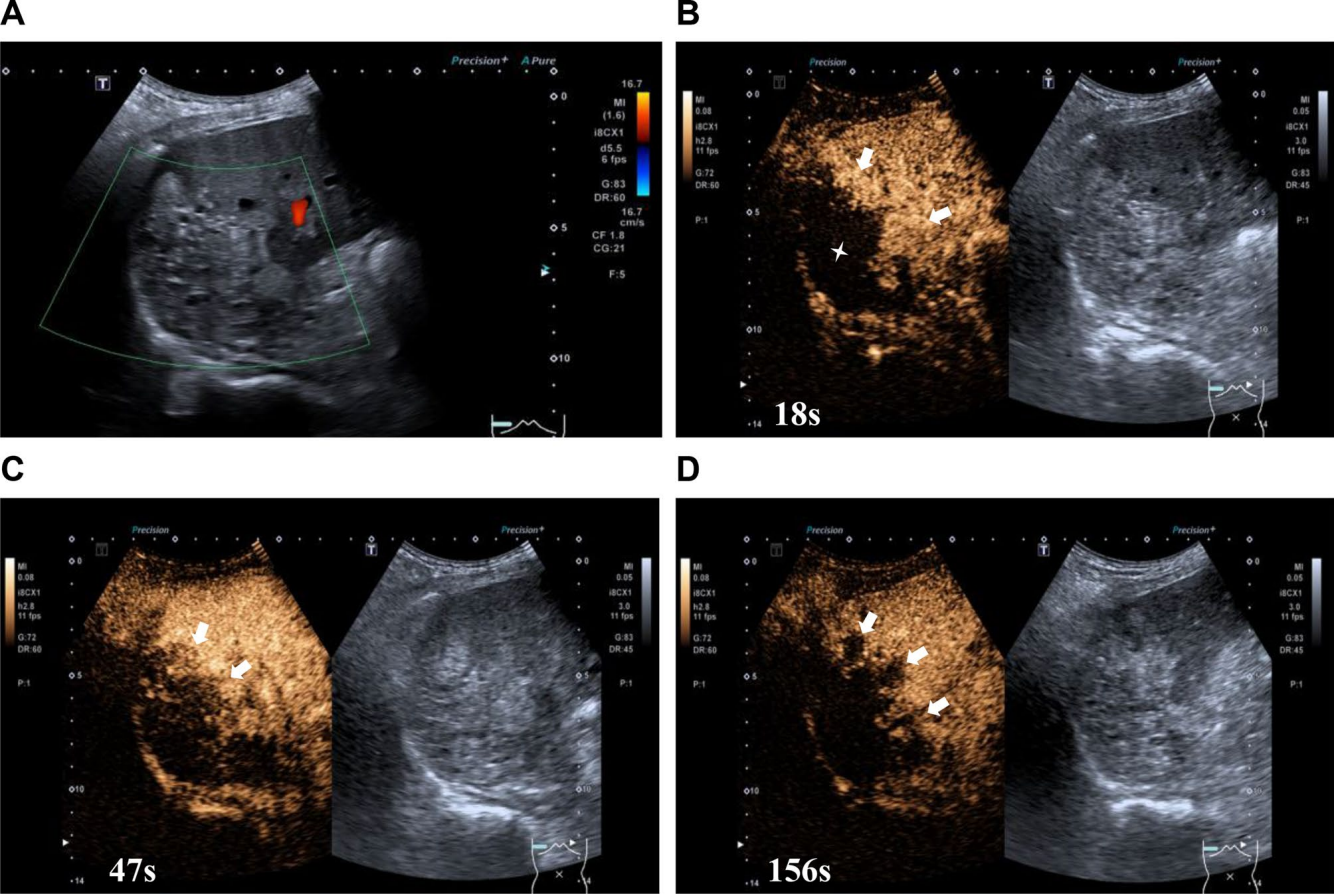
Ultrasound findings. (A) Conventional ultrasound revealed a heterogeneous mass in the right lobe of the liver. (B) Contrast-enhanced ultrasound showed the periphery of the lesion was slightly hyper-enhanced compared to the surrounding normal liver tissue at 18 s after contrast agent injection (arrow), with a blurred boundary and no enhancement in the interior of the lesion (asterisk). (C) Contrast-enhance ultrasound at 47s after contrast agent injection revealed that the contrast agent began to wash out at the periphery of the lesion (arrow). (D) Contrast-enhance ultrasound at 156s after contrast agent injection showed the periphery of the lesion was hypo-enhancement (arrow) and no enhancement in the inner part
